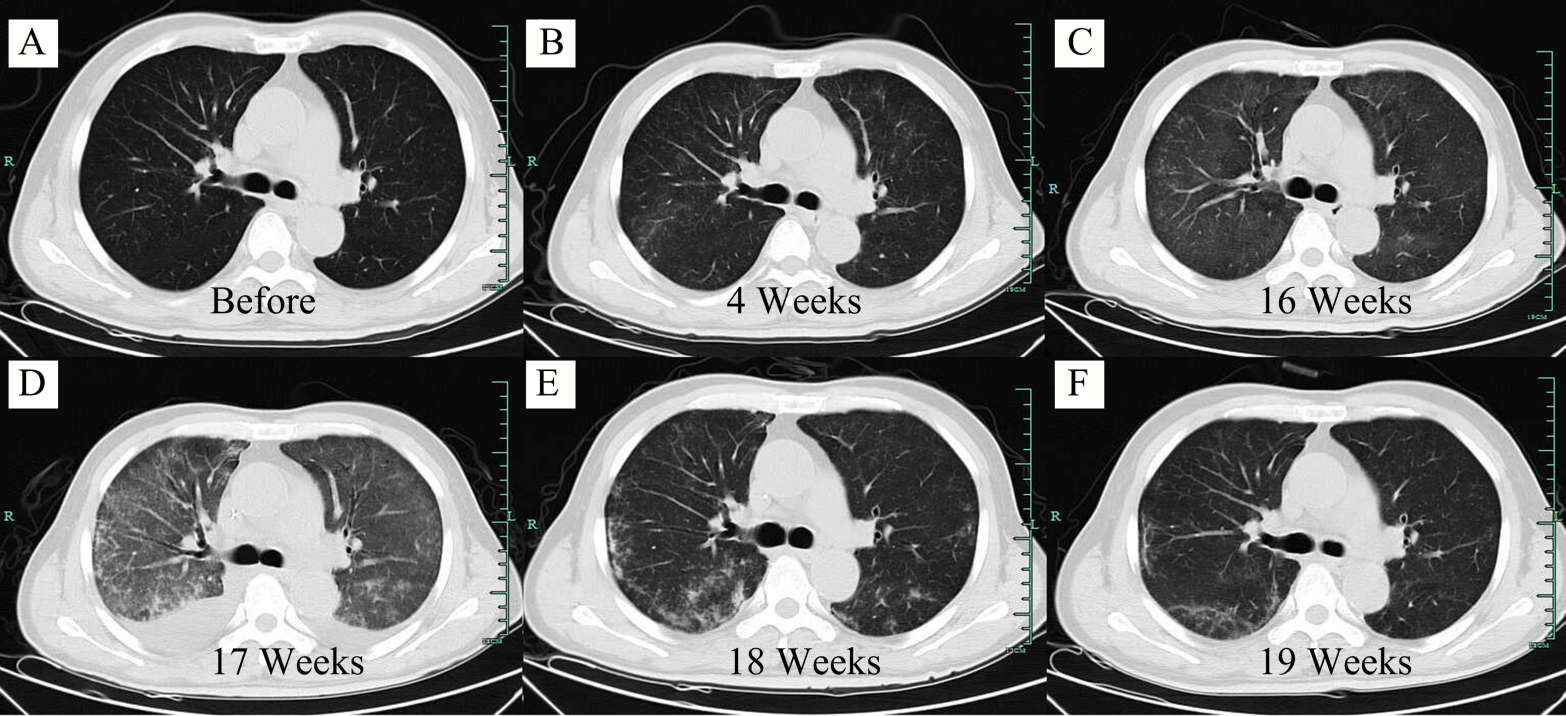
Axial computed tomography (CT) scan of the lung at different time points. (A) Screening period before CAR-T cell infusion. (B) 4 weeks after CAR-T cell infusion. (C) 16 weeks after CAR-T cell infusion. (D) 17 weeks after CAR-T cell infusion. (E) 18 weeks after CAR-T cell infusion. (F) 19 weeks after CAR-T cell infusion.
